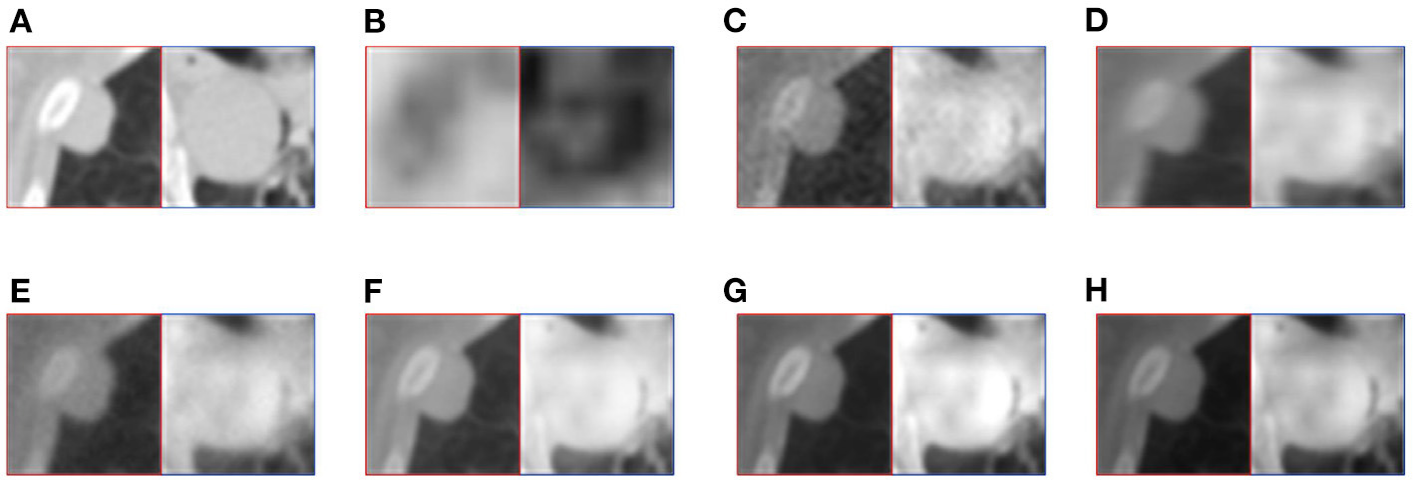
The detail of fusion results of patient B. (A) CT; (B) PET; (C) AD; (D) GF; (E) PAPCNN; (F) MCFNET; (G) IFCNN; (H) OURS.
